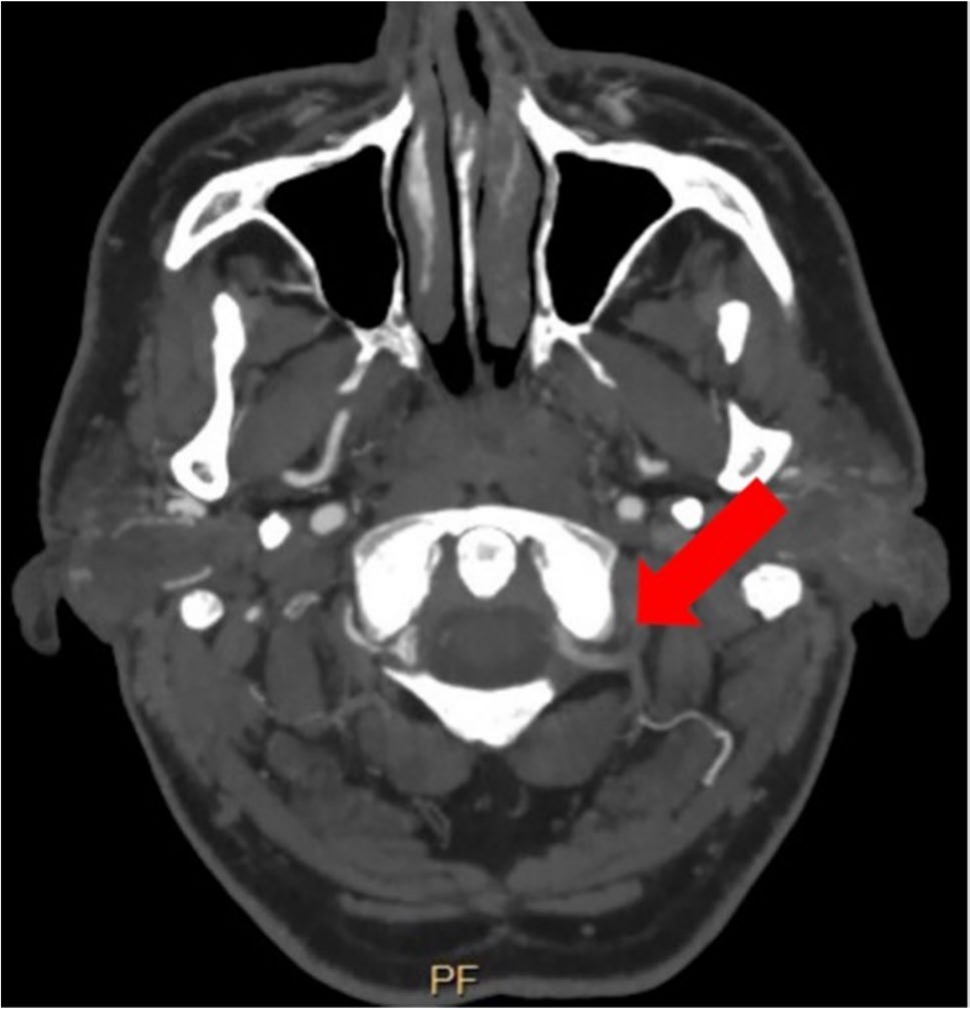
CT angiography proving good patency of V3 segment on the left side
